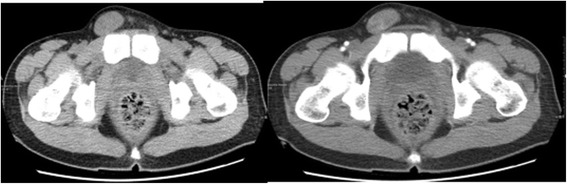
The CT scan showing cryptorchidism with peripheral enhancement in the right groin area
